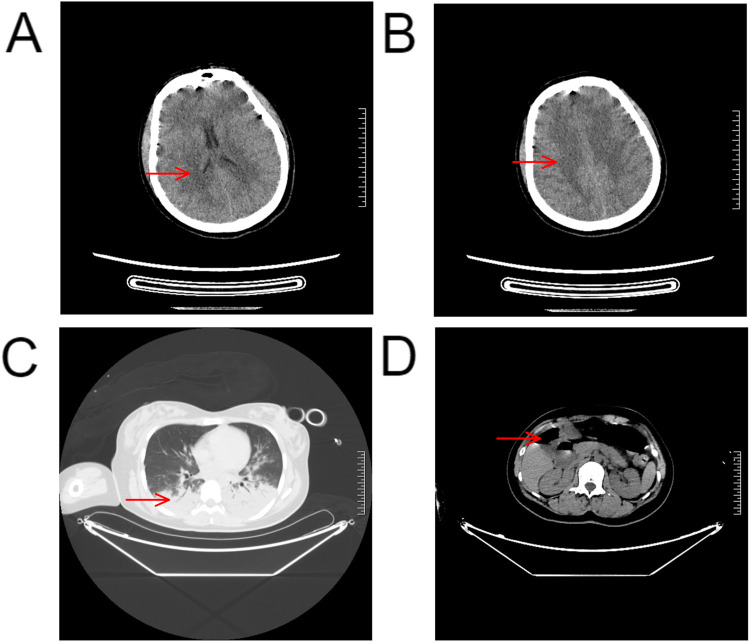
Plain CT scan of the head, chest, and abdomen of a 17-year-old girl on the day of admission. Both (A) and (B) exhibit blurred sulci and fissures in the bilateral cerebral hemispheres, diffuse edema of the brain tissue. (A) Red arrow indicates a symmetrical reduction in white matter density adjacent to the lateral ventricle. (B) Red arrow indicates a decrease in white matter density within the semi-oval center of the brain. (C) Red arrow points to exudation and consolidation in lung. (D) Red arrow shows intestinal dilation with accumulation of gas and fluid.
